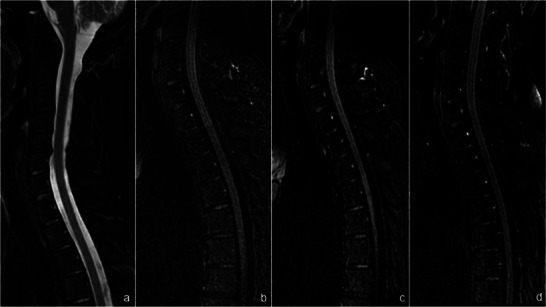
Presentation of a case without visible cerebrospinal fluid (CSF) lamella on either noncontrast MR myelography or contrast‐enhanced heavily T2‐weighted fluid‐attenuated inversion recovery (ceHT2‐FLAIR) MRI. A 47‐year‐old female patient with intracranial hypotension (case #8) presented with orthostatic headache and nausea upon standing, beginning 7 weeks after lumbar puncture. In the sagittal modified DIXON T2 sequence (a), no signs of a CSF leak were observed; therefore, no axial plane was acquired. Sagittal noncontrast HT2‐FLAIR (b) as well as postcontrast HT2‐FLAIR imaging at 10 min (c) and 3 h postinjection (d) confirmed the absence of a CSF lamella. CT myelography was deemed unnecessary, and the patient was treated with a nontargeted epidural blood patch.
